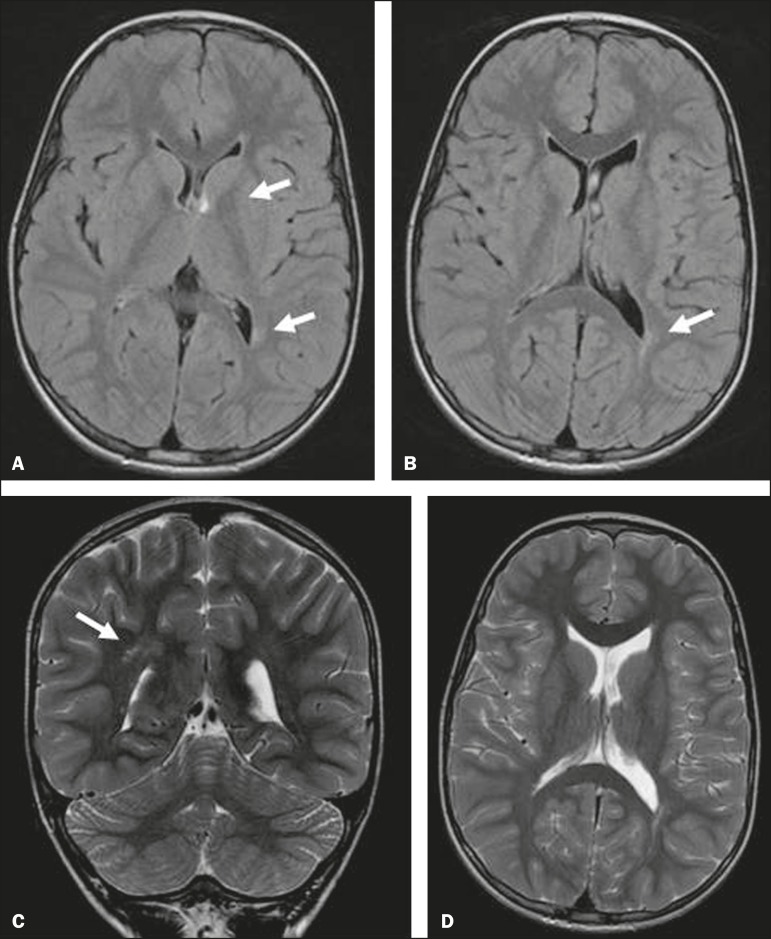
MRI of the brain showing ventricular asymmetry (left lateral ventricle
